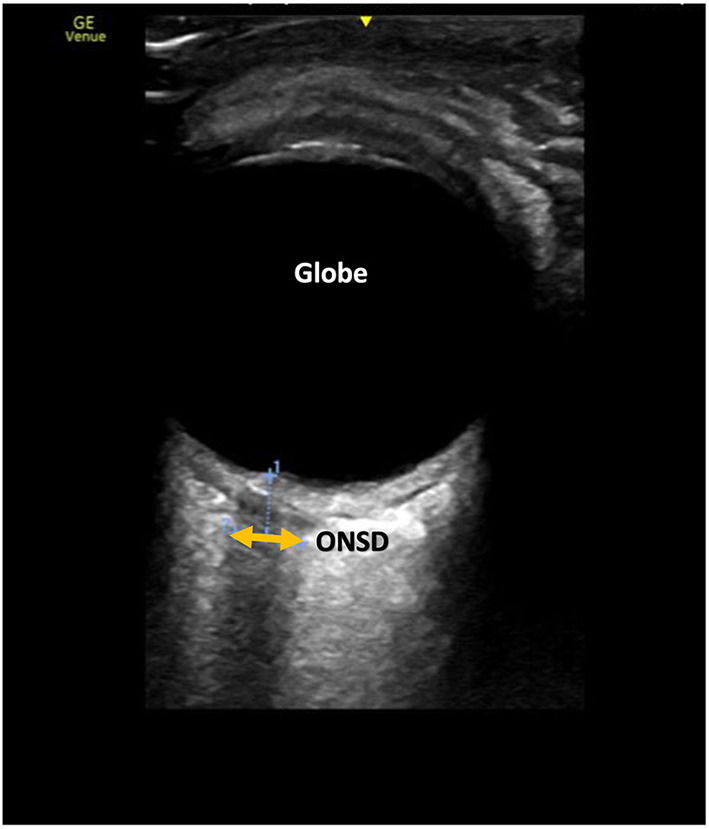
Ocular ultrasound demonstrating optic nerve sheath diameter measurement (ONSD). The sheath appears as a less bright (hypoechoic) structure compared to the surrounding tissue.
